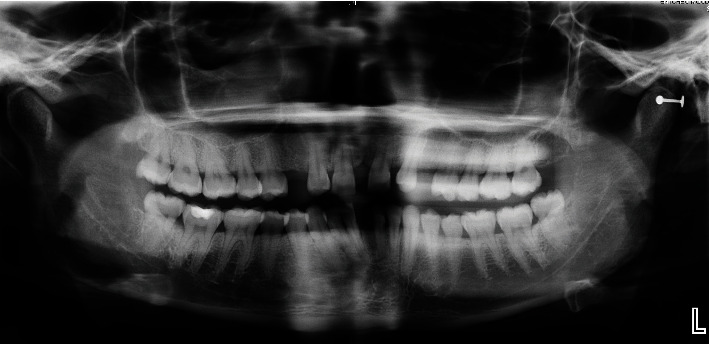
Initial panoramic radiography.
